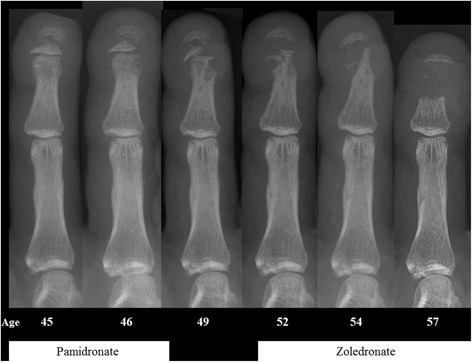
Progression of acro-osteolysis despite treatment with bisphosphonates. Radiographs of the right index finger of subject 7, taken over 12 years. The timing of bisphosphonate treatment is indicated
